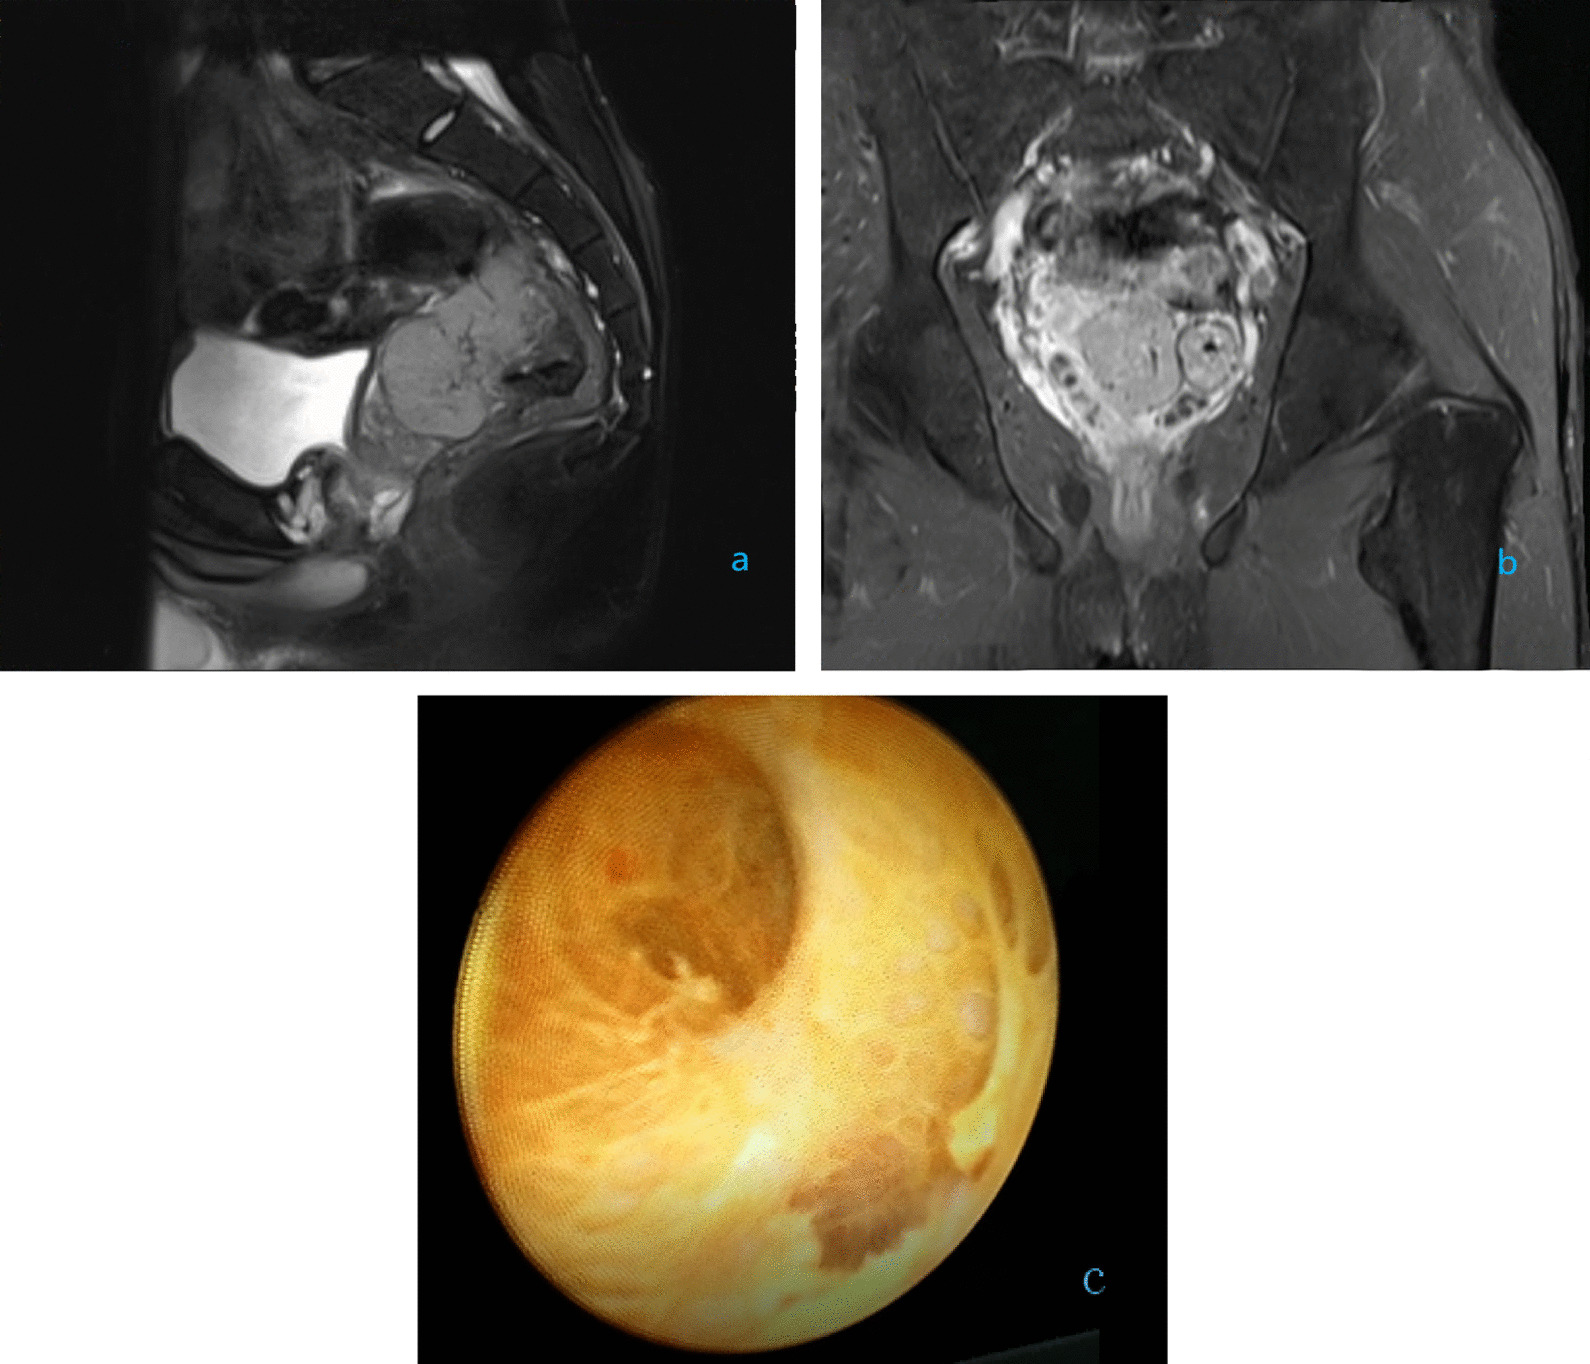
a, b Pelvic MRI plain scan + DWI + enhancement indicated abnormal signal above the posterior to the seminal vesicle, which was closely related to the seminal vesicle; c transurethral seminal vesiculoscopy revealed chronic inflammatory changes in the right seminal vesicle
